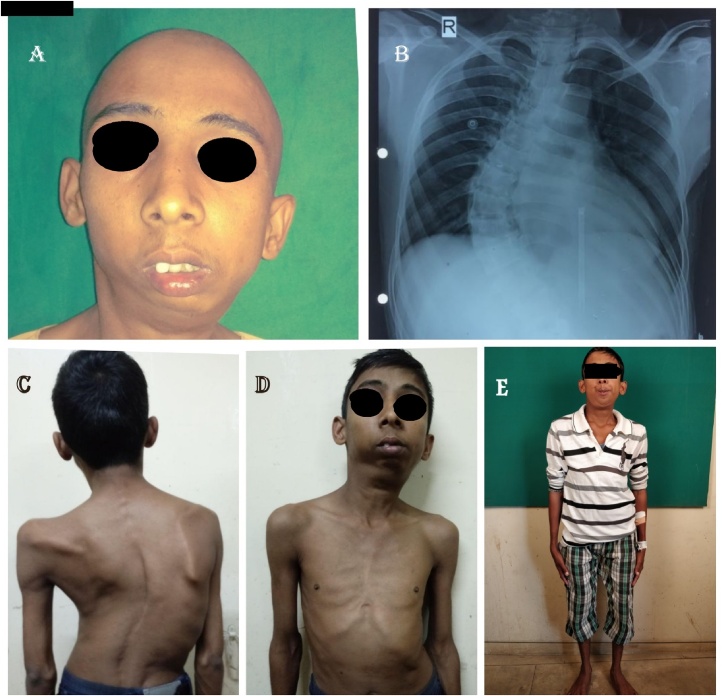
Pre-operative Photographs. A: Frontal Profile, B: Chest Radiograph, C,D: Skeletal Features, E: Full Body Photograph.
